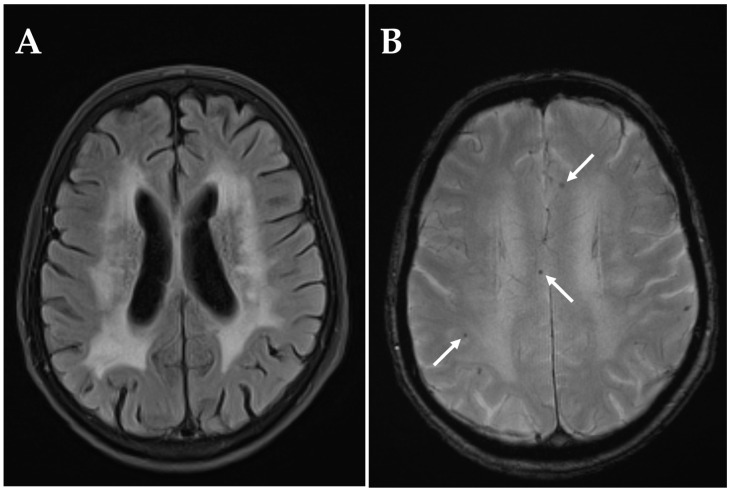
MRI indications of cerebral small vessel disease in a patient with NAION and presumably microvascular stroke. (A) Fluid-attenuated inversion recovery (FLAIR) sequence showing leukoaraiosis. (B) T2* sequence revealing several cortical microbleeds (arrows).
